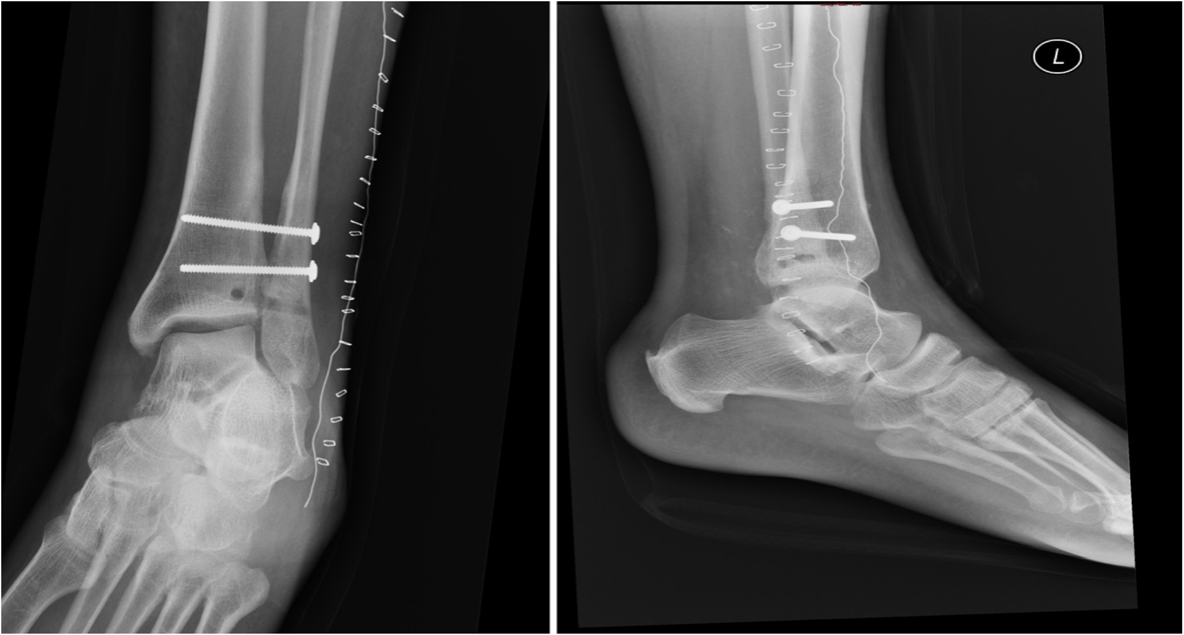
Postoperative radiograph at day 1. An internal rotation (mortise) and lateral view radiographs of the left ankle showing syndesmotic position and fixation. The tibiofibular osseous canals are also visible inferior to the bottom screw
